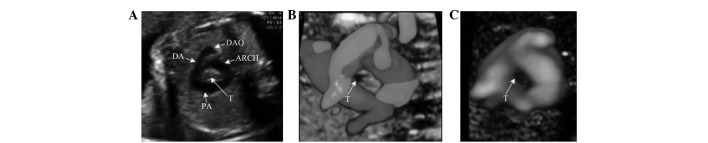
Case of a vascular ring in a 27-week fetus diagnosed by echocardiography. Vascular ring indicated by (A) 2D echocardiography; (B) in vitreous volume imaging indicated by STIC combined with color Doppler; and (C) in volume imaging indicated by STIC combined with power Doppler. T, trachea; PA, pulmonary artery; DA, ductus arteriosus; DAO, descending aorta; ARCH, aortic arch.
